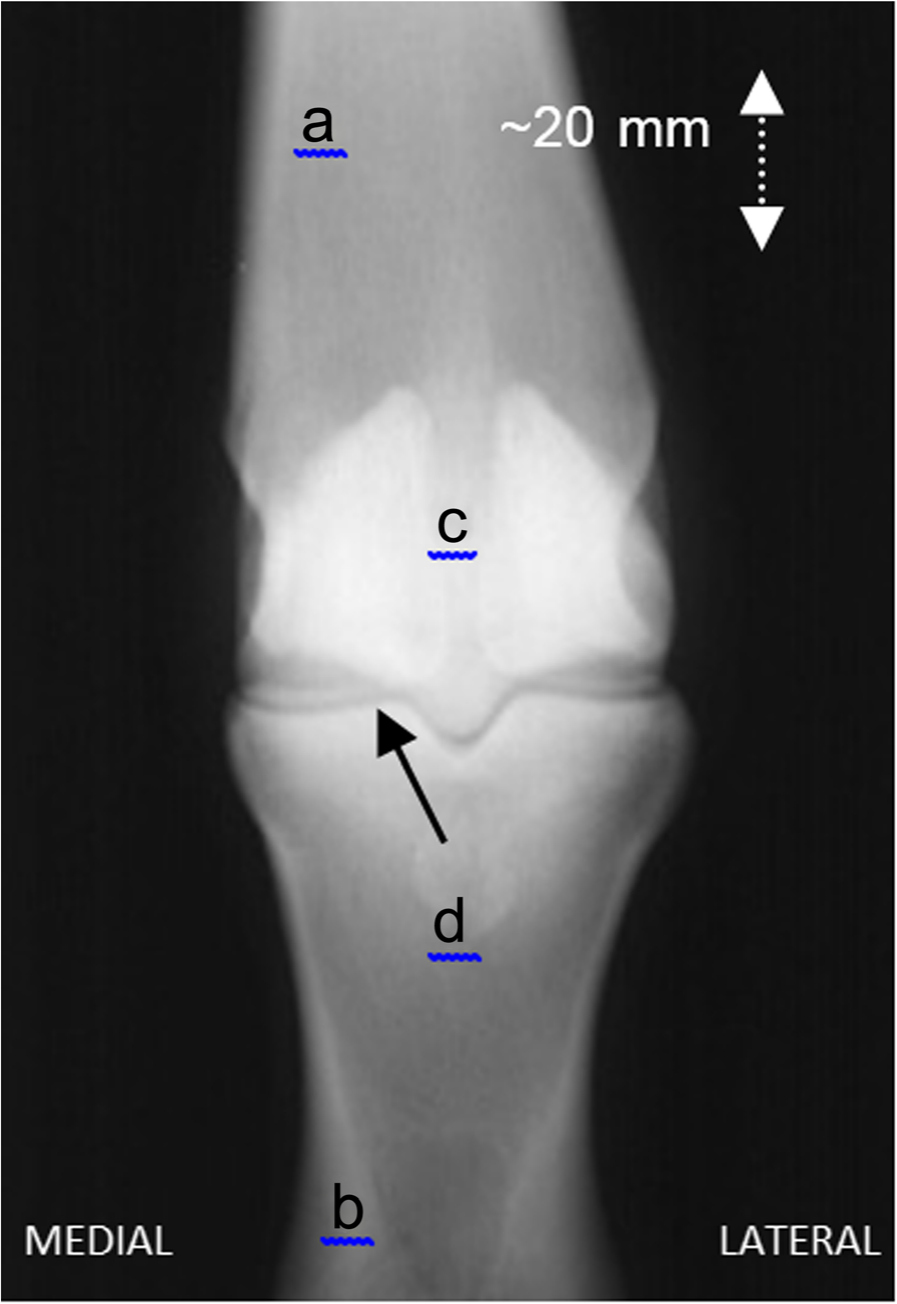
Dorso-palmar (antero-posterior) radiograph of the metacarpophalangeal joint. Key: a third metacarpal bone, b proximal phalanx, c proximal sesamoid bones, d metacarpophalangeal joint
